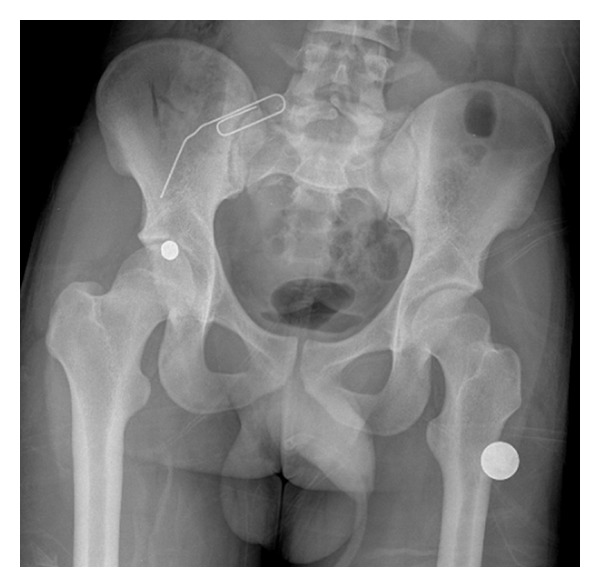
AP pelvis radiograph showing the bullet in the right femoral head. The tip of the paper clip shows the entry wound.
